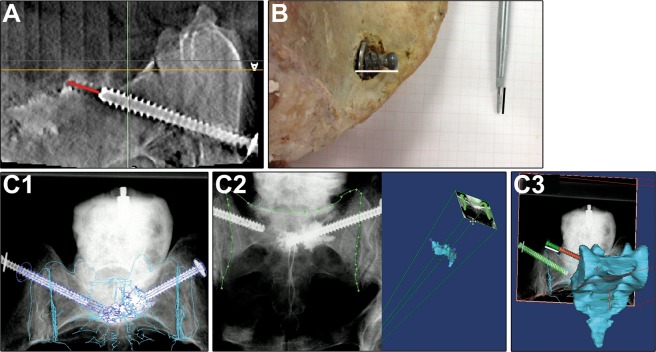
Measurement of SI screw loosening using the 3D scan (A), optical measurement (B) and the X-ray module (C), n = 9. (A) SI screw turn back (red line) indicated by the radiolucency was measured in the 3D scan. (B) Optical measurement: A K-wire within a drill sleeve was applied parallel to the SI screw. After retraction of the drill sleeve to the screw head, the length of the extruding K-wire was assessed after placing on millimetre paper using ImageJ (black line). (C) X ray module: A 3D model based on the CT scan was generated using Mimics and each fluoroscopic image (AP, outlet, inlet) of T_1_ was manually registered to the beforehand-created 3D model (C1). The registration was further enhanced by contour-based registration (C2). One screw model (in red) was adjusted to the screw position of the CT scan, another screw model (green) was positioned according to the fluoroscopic images of T_1_ (green screw). The distance between these screws (white line) was measured.
